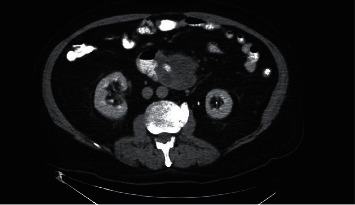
Initial CT scan showing mass in the duodenum.
